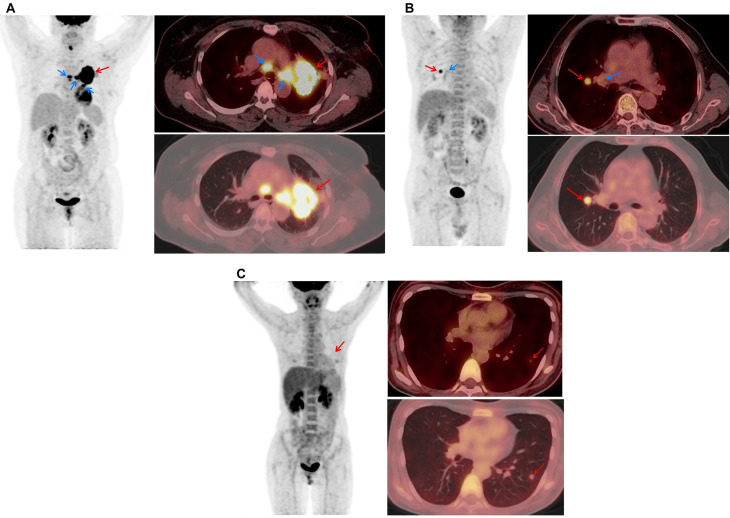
(A) A representative case of a patient with PET/CT-positive lymph nodes and pathologically confirmed LNM (true-positive). (B) A representative case of radiographically occult LNM (OLNM), where lymph nodes were PET/CT-negative but pathologically proven to harbor metastasis (false-negative). (C) A representative case of a patient with PET/CT-negative lymph nodes and pathologically confirmed absence of LNM (true-negative). The red arrows point to the primary tumor, and the blue arrows point to the metastatic lymph nodes
